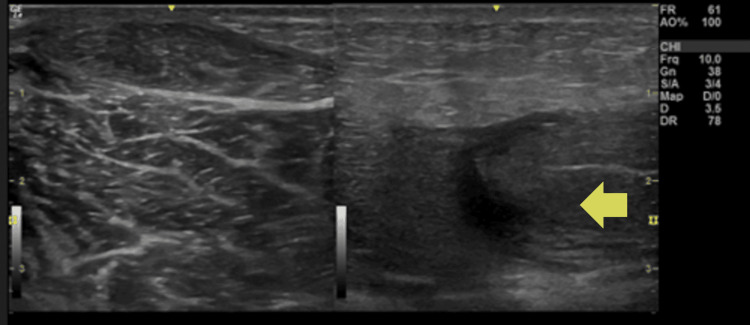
POCUS findings: the left normal thigh muscles and right edematous thigh muscles. POCUS: point-of-care ultrasound.
